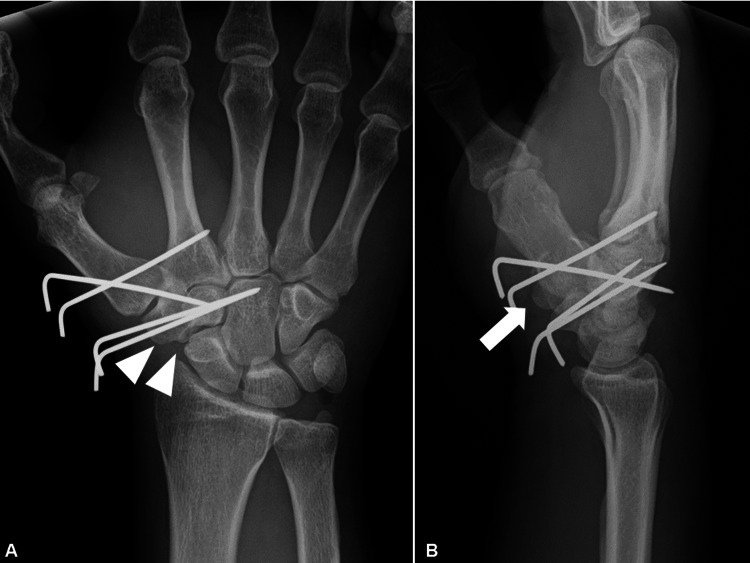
Postoperative plain radiography. (A) Anteroposterior and (B) lateral views of the right wrist (arrowheads show reduction of the trapezium, and arrow shows reduction of the volar fragment of the trapezium).
